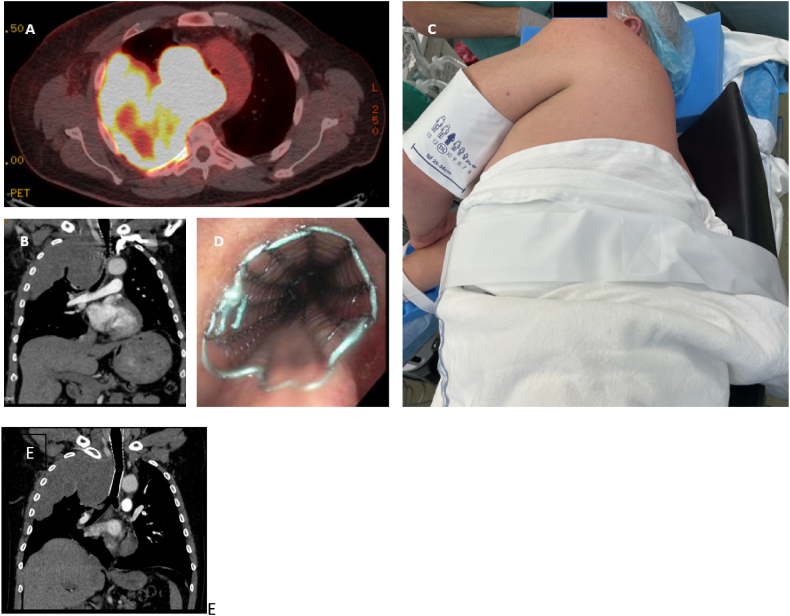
A. Positron Emission Tomography (PET) of heterogeneously enhancing right-sided pleural-based masses invading adjacent mediastinum and compressing adjacent mid trachea, esophagus, and superior vena cava; B. Computed tomography (CT) sagittal view demonstrating severe mid tracheal compression; C. Patient in right lateral semi-seated position for rigid bronchoscopy; D. 16 × 60°mm Ultraflex metallic stent deployed in the distal trachea; E. Post procedural CTA with patent tracheal stent.
